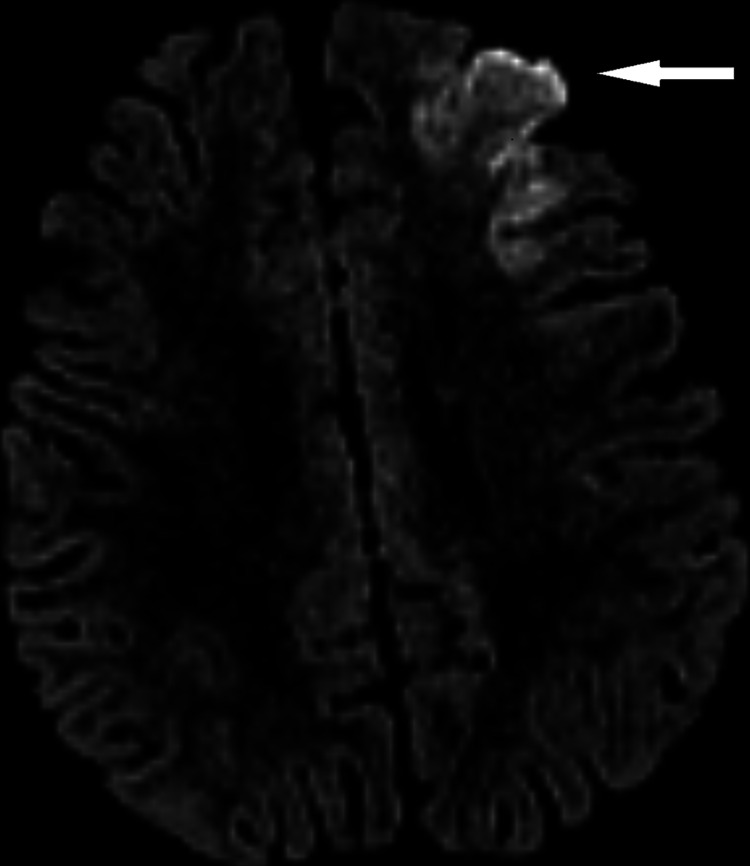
MRI shows left frontal cortical and subcortical area of restricted diffusion consistent with acute infarct.
